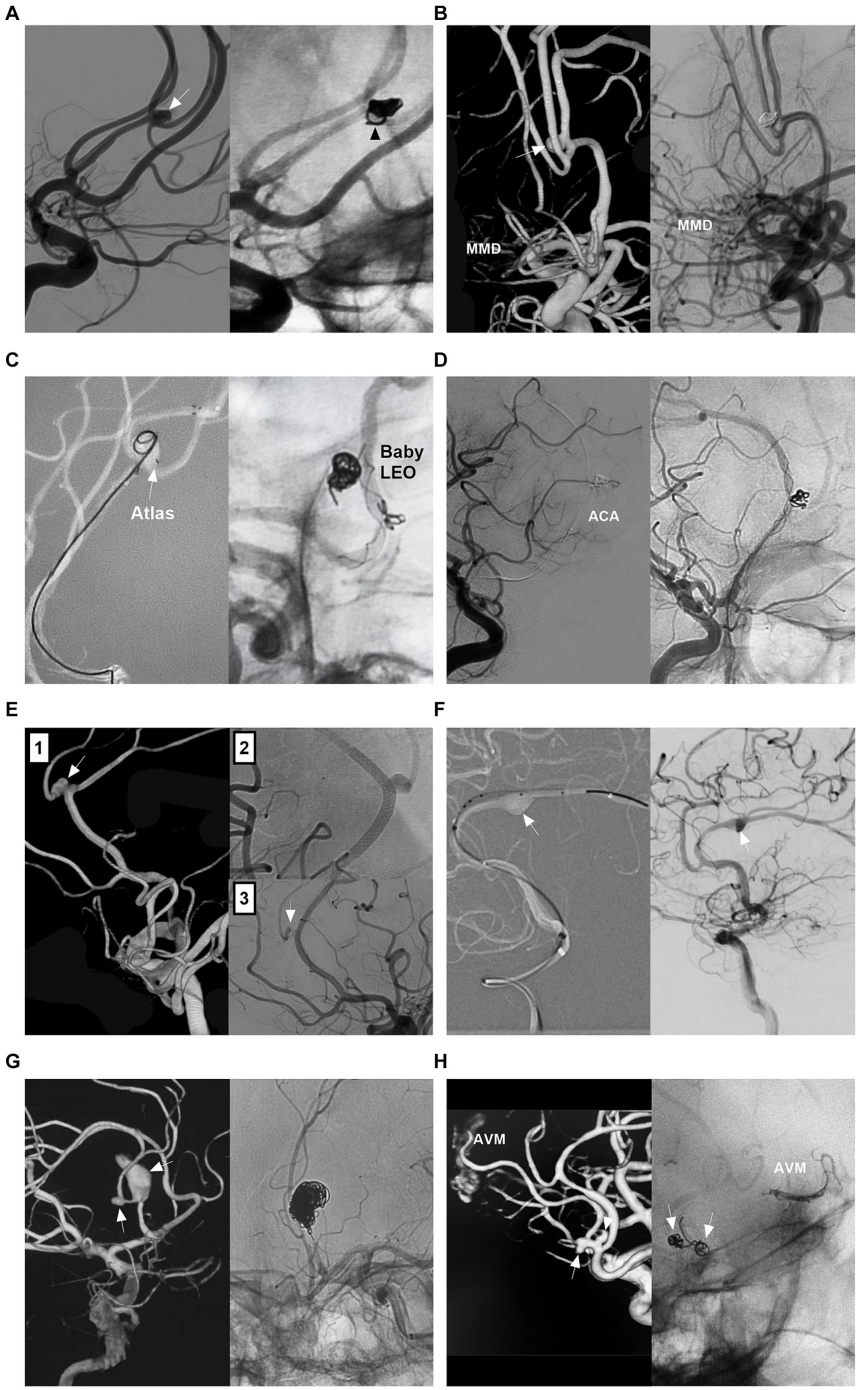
Treatment of distal ACA aneurysms. (A) Left panel: DSA showing a distal ACA aneurysm (arrow); Right panel: DSA showing that the aneurysm was coiled completely; one coil loop (arrowhead) provided support without stent assistance. (B) Left panel: 3D-DSA showing a distal ACA sidewall aneurysm (arrow) with MMD; Right panel: DSA showing that the aneurysm was coiled completely. (C) Left panel: Roadmap image showing a distal ACA wide-necked aneurysm (arrow) was coiled with Atlas stent assistance, the tail of the stent (arrow) was deployed in the aneurysm; Right panel: Unsubtracted DSA showing a small ACA aneurysm above the AcomA was coiled with baby LEO stent assistance. (D) Left panel: DSA showing the occlusion of the ACA after the catheterization of double microcatheters; Right panel: Unsubtracted DSA showing the ACA recanalization after removing the microcatheters. (E) Panel 1: 3D-DSA showing a distal ACA aneurysm with a branch incorporation (arrow); Panel 2: panel: DSA showing the flow diverter covering the aneurysm; Panel 3: Follow-up DSA showing the aneurysm regressed. (F) Left panel: Roadmap image showing that a distal ACA dissecting aneurysm (arrow) was being covered by a flow diverter; Right panel: DSA showing the contrast agent retention (arrow) in the aneurysm. (G) Left panel: 3D-DSA showing two tandem A2 aneurysms (arrows); Right panel: Unsubtracted DSA showing that the proximal large aneurysm together with proximal parent artery was occluded. (H) Left panel: 3D-DSA showing two tandem aneurysms (arrows) at the origin of the feeding artery to an AVM; Right panel: Unsubtracted DSA showing that the aneurysms (arrows) were coiled, and the AVM was embolized by Onyx. 3D, three-dimensional; ACA, anterior cerebral artery; AVM, arteriovenous malformation; CTA, computed tomography angiography; DSA, digital subtracted angiography; MMD, moyamoya disease.
